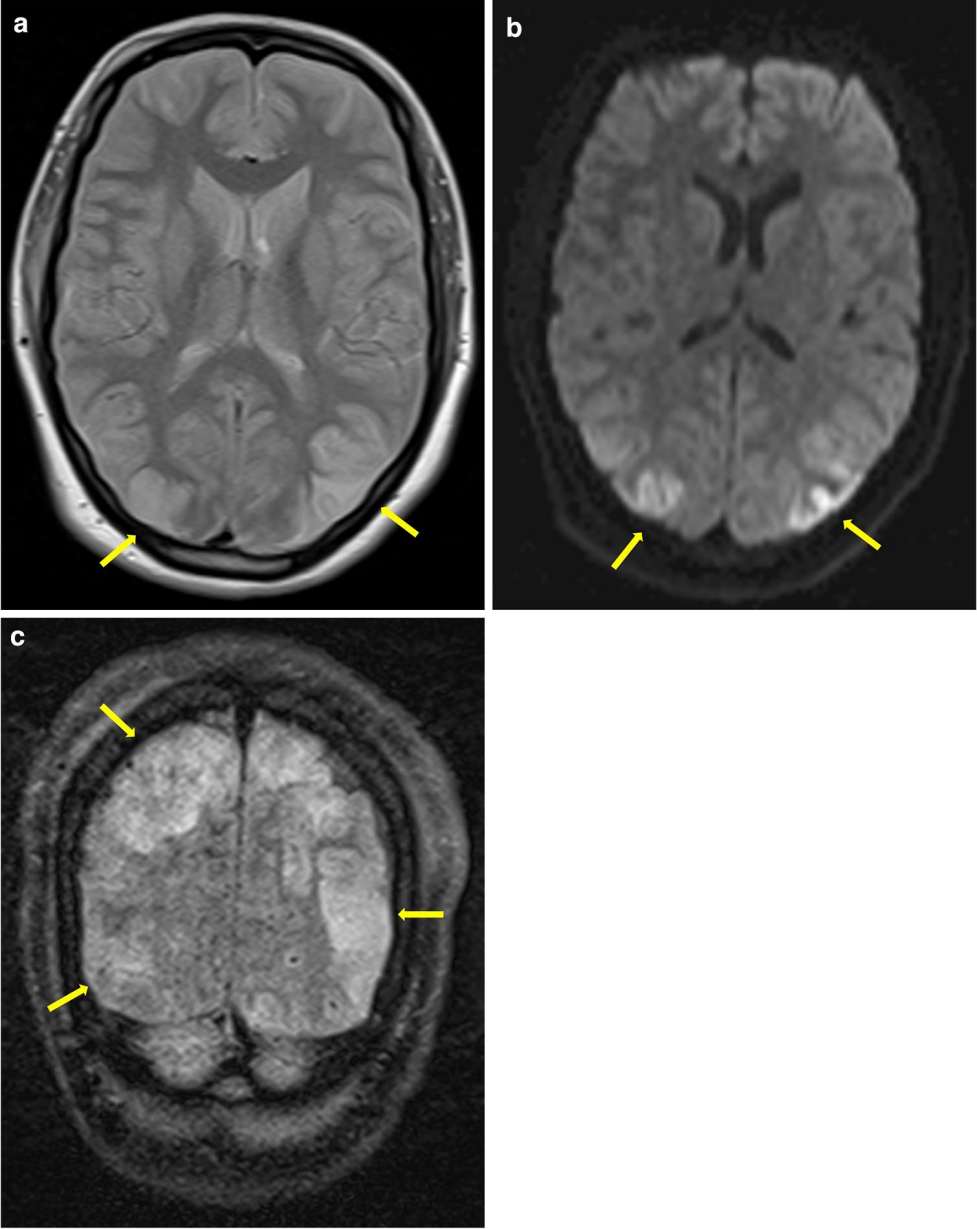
This figure represents the characteristic MRI findings of posterior eversible encephalopathy syndrome (PRES), one of the most common complications of reversible cerebral vasoconstriction syndrome (RCVS). a–c Bi-occipital hyperintensities in different T2-weighted images without ADC (apparent diffusion coefficient) hypointensities, corresponding to PRES (yellow arrows). a Proton-density-weighted (PDw) MRI in the transverse plane, b DWI in the transverse plane, and c FLAIR in the coronal plane
